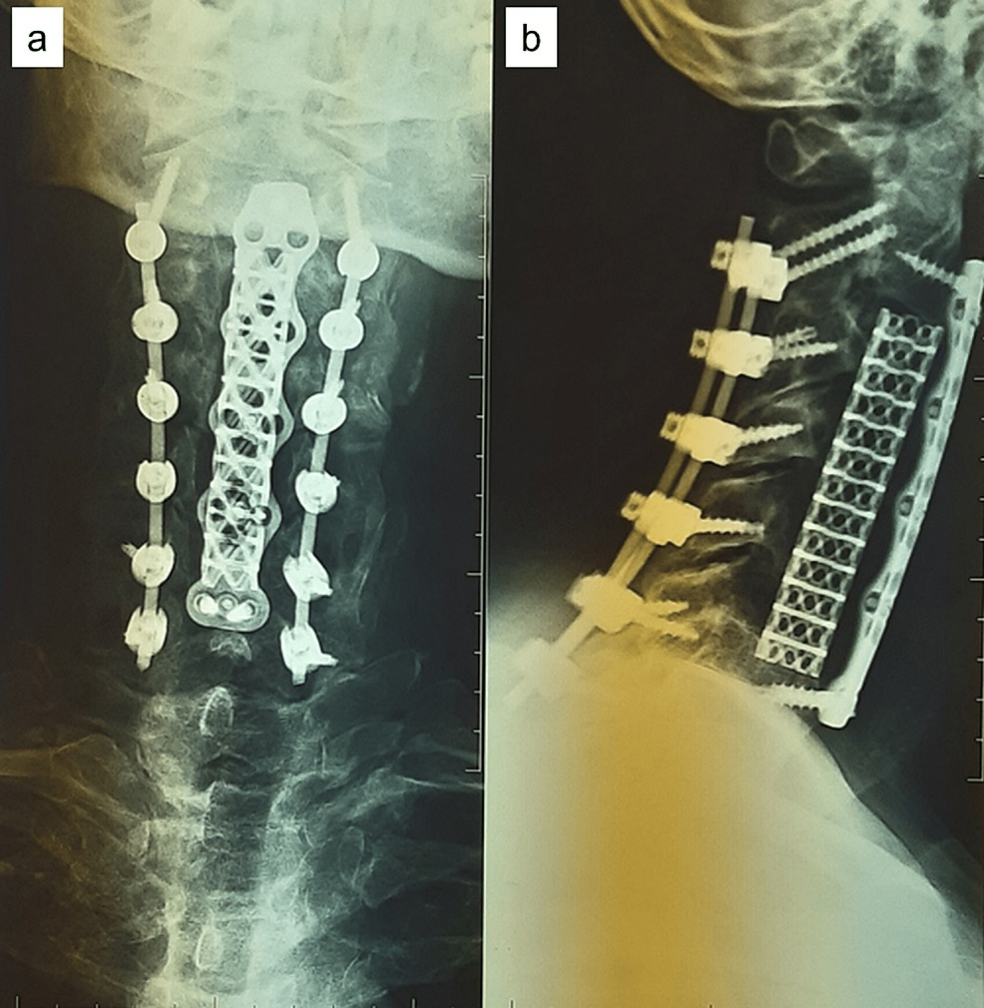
Postoperative X-ray Cervical plain radiographs show a titanium mesh at the site of corpectomy C3-C5 secured by an anterior plate as well as a posterior pedicle, lateral mass screws and rods C2-C7: (a) anteroposterior, (b) lateral
